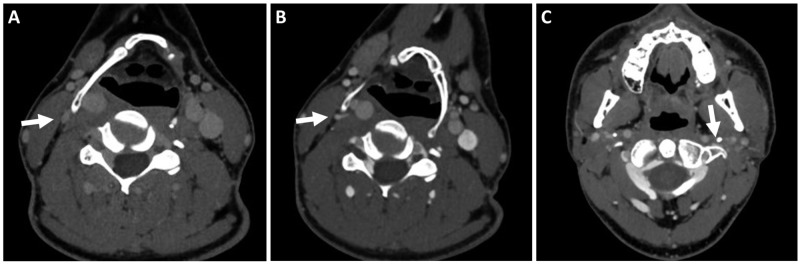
Preoperative axial CTV. (A, B) preoperative axial CTV images showing compression of the right IJV (white arrows) by the hyoid bone in (A) neutral head position and worsening compression on (B) left head turn. (C) Axial CTV demonstrating compression (white arrow) of the contralateral distal IJV between the styloid process and transverse process of first cervical vertebrae. CTV, computed tomography venogram; IJV, internal jugular vein
